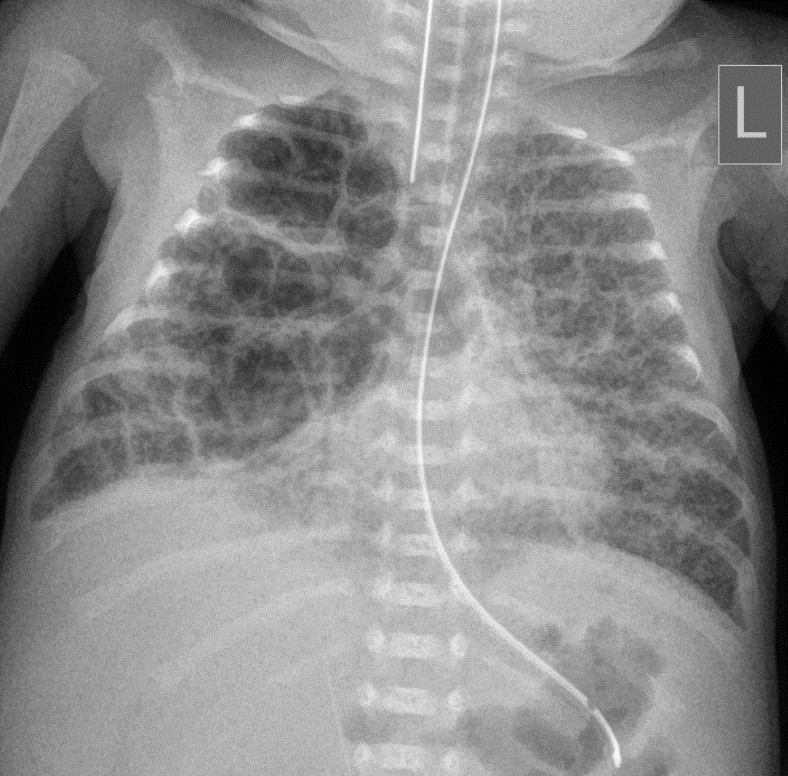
AP chest radiograph on the 46^th^ day of life (8 days after fibrin glue application) shows resolution of right pneumothorax (drain was removed). Marked hyperinflation with coarse interstitial markings and cystic changes in the right lung; signs of PIE in the left lung.
